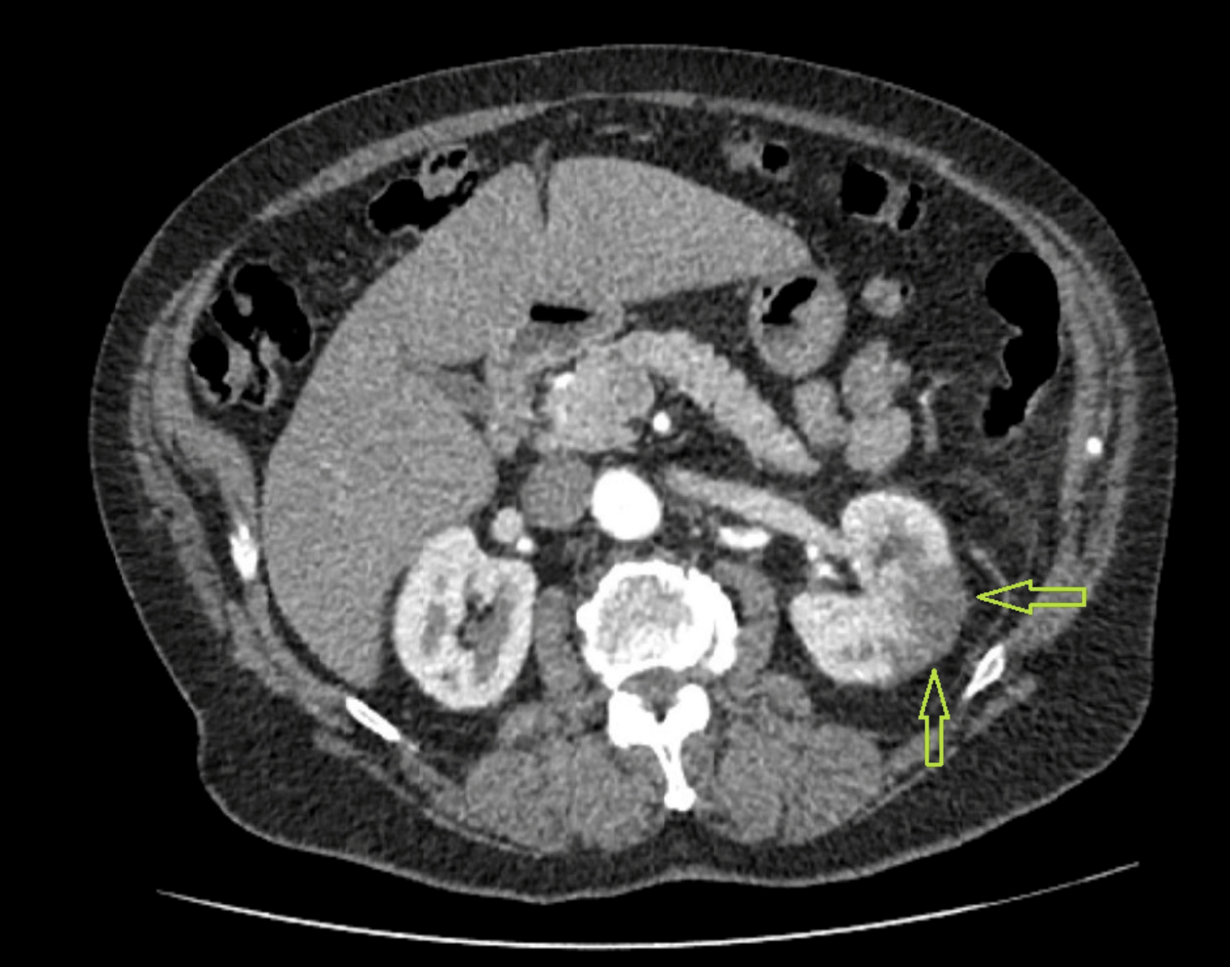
CT angiography of the abdomen and pelvis with IV contrast (contrast-enhanced CT) showing left renal infarct. Arrows showing a wedge-shaped area of decreased attenuation in the left kidney, which is indicative of a renal infarct.
